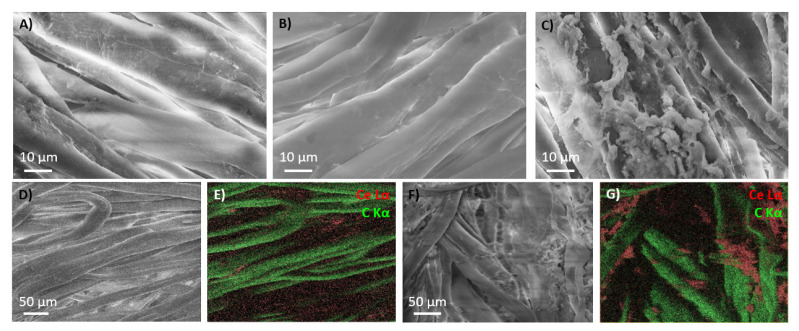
SEM images of the textile after ultrasonic treatment in the cellulose dispersion (A), the nonmodified CeO_2_ sol (B) and the CeO_2_/cellulose sol (C). SEM images and the corresponding cerium- and carbon-distribution EDS maps of the textile after ultrasonic treatment in nonmodified CeO_2_ sol (D,E) and CeO_2_/cellulose sol (F,G).
